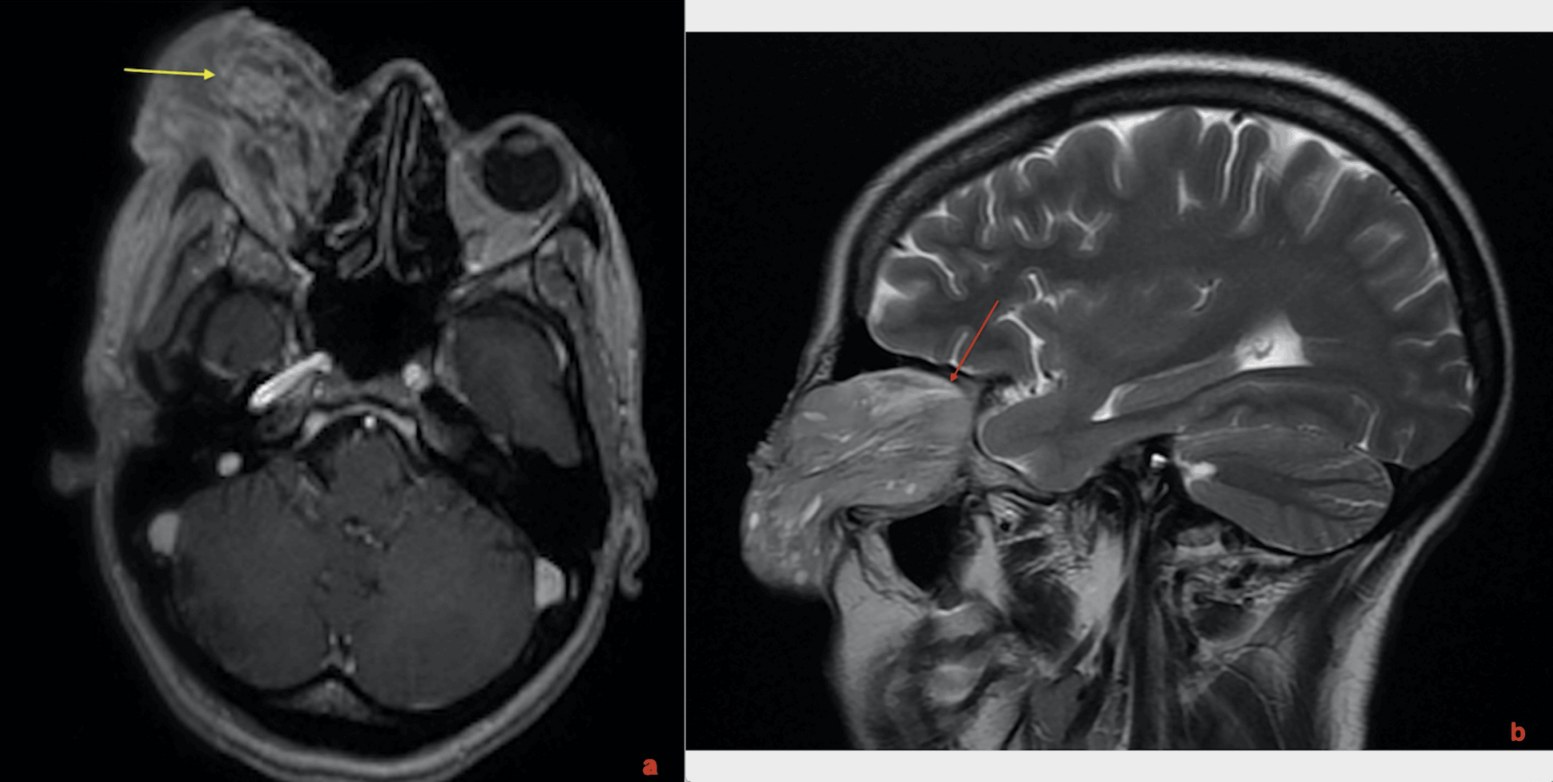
Orbital and cranial MRI showing a large mass involving the globe, muscles, lacrimal gland, and extraconal fat (yellow arrow in figure (a)), with initial intracranial infiltration (red arrow in figure (b)).
